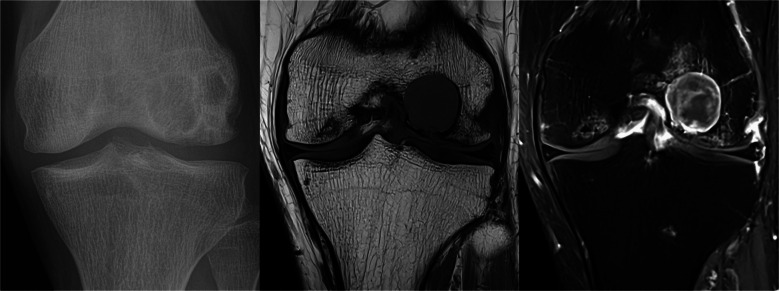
Twenty-five-year-old male with subchondral cyst. AP radiograph of the knee (left) with lateral tibiofemoral compartment osteoarthritis and subarticular cystic change underlying the lateral femoral condyle subchondral bone plate. Coronal T1-weighted image (centre) shows well-defined cystic signal characteristics underlying focal distortion of the subchondral bone plate. Incidental note is made of osteonecrosis involving the medial femoral condyle which demonstrates central fat signal. Coronal T1FS post-contrast image (right) highlighting predominantly thin peripheral enhancement
